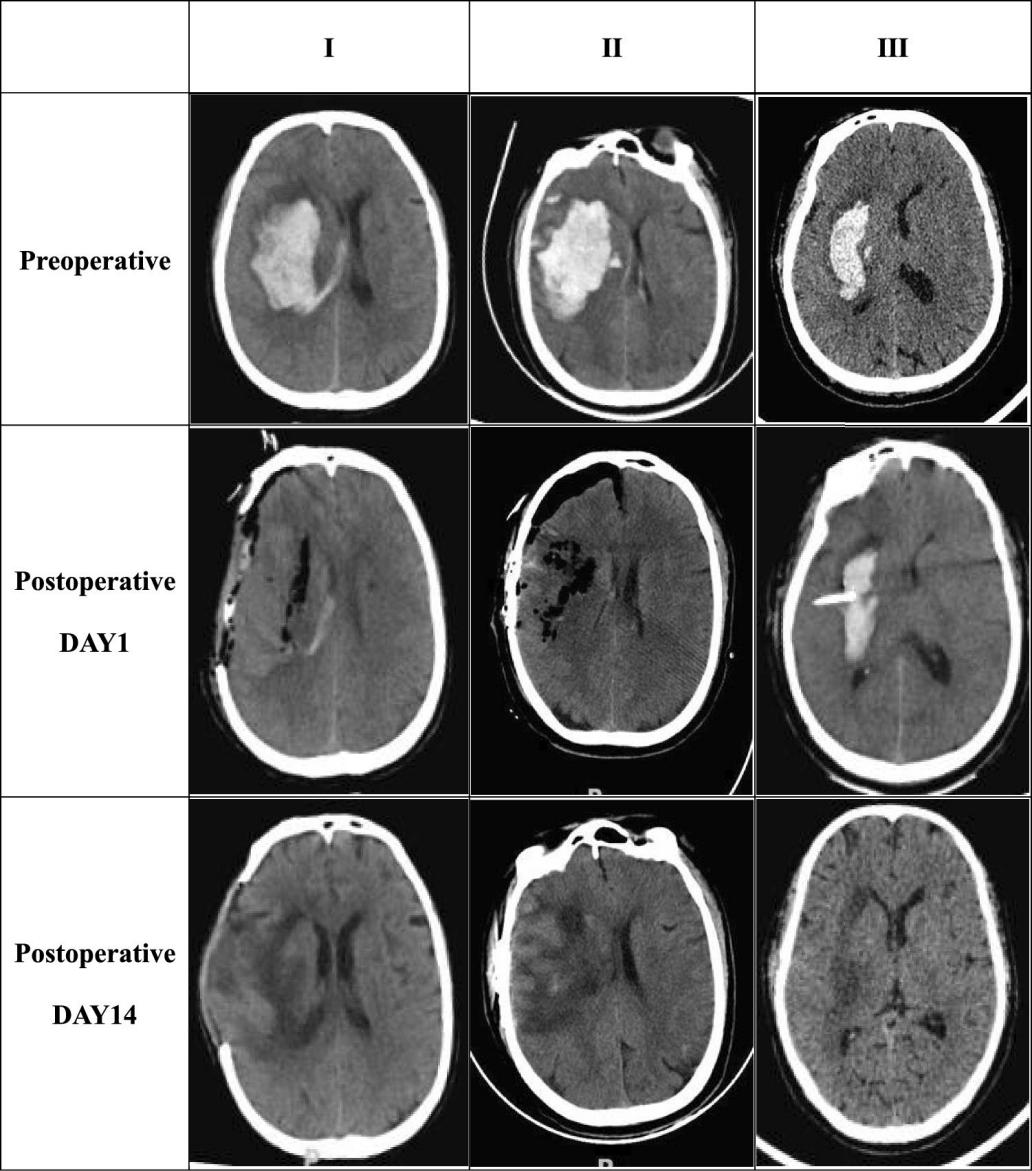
CT manifestations of different surgical approaches in the right BGH.
